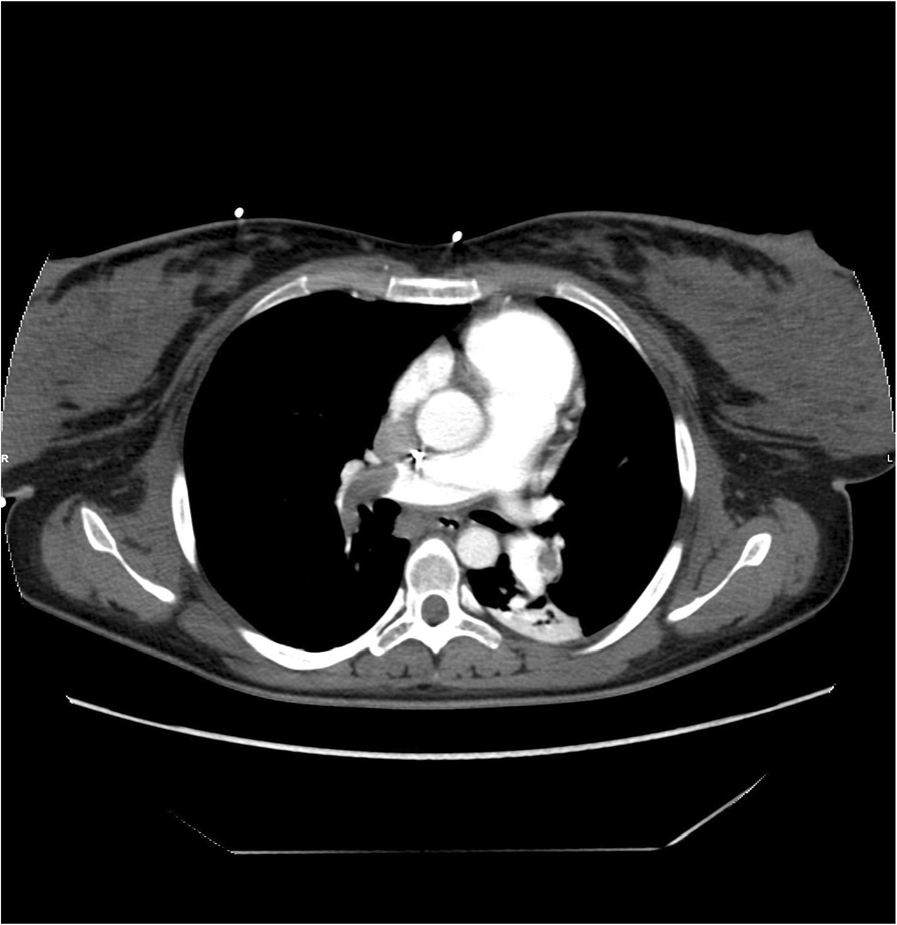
Pulmonary CT angiogram. The CT angiogram shows many intraluminal filling defects suggestive of massive bilateral pulmonary embolism
